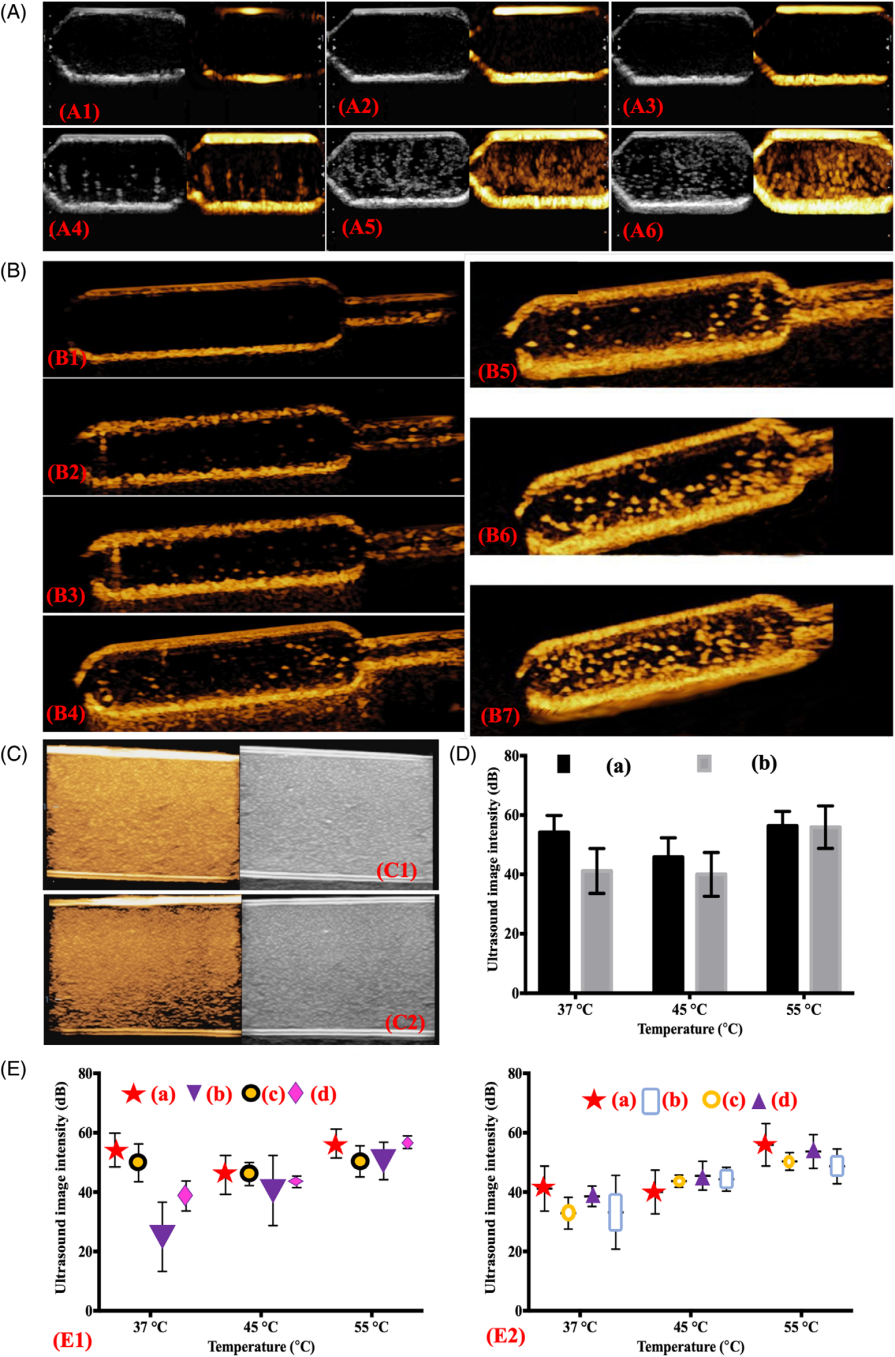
(A) Dual‐targeted theragnostic ultrasound contrast agent (DTTUCA) nanodroplets produced ultrasound (US)‐enhanced images under CEUS and B model. The operational fundamental frequency was 7.5 MHz, and the MI values were 0.2, 0.4, 0.6, 0.8, 1.0, 1.2 (from A1 to A6). (B) DTTUCA nanodroplets promoted US‐enhanced images under CEUS model. The operational fundamental frequency was 13 MHz, and the MI values were 0.08, 0.14, 0.20, 0.30, 0.40, 0.60 and 0.80 (from B1 to B7). (C) DTTUCA nanodroplets produced US‐enhanced images with CEUS and B model. The operational fundamental frequencies were 15 MHz (C1) and 20 MHz (C2), and the MI values were 0.2 (C1) and 0.14 (C2). (D) Quantitative analysis for ROI US images intensity of DTTUCA nanodroplets under different temperatures: fundamental frequencies were 15 MHz (a) and 20 MHz (b), and the MI values were 0.2 (a) and 0.14 (b). (E) Comparison of ROI US images intensity under different temperatures for DTTUCA nanodroplets (a), untargeted mPEG_2k_‐PCL nanodroplets (b), cRGD‐PEG_2k_‐PCL nanodroplets (c) and untargeted mPEG_2k_‐PCL@DOX nanodroplets (d). The fundamental frequencies were 15 MHz (E1) and 20 MHz (E2), and the MI values were 0.21 (E1) and 0.14 (E2)
